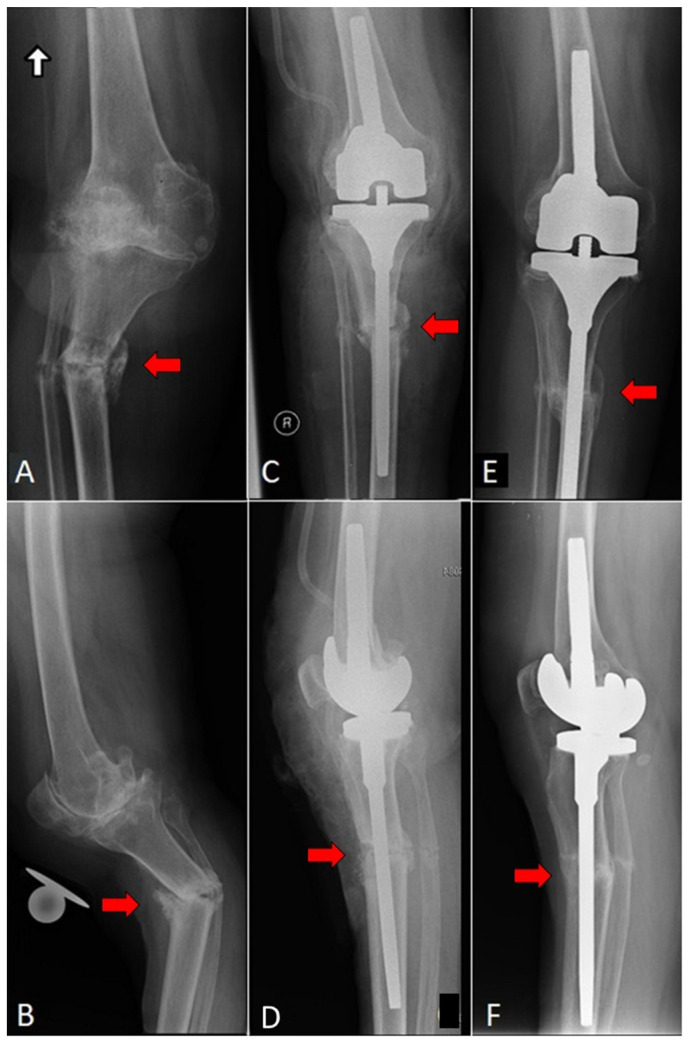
(A) AP and (B) lateral radiograph of the patient with 1/3 proximal tibial and fibular shaft fractures (pseudarthrosis) and coexisting severe knee osteoarthritis. Early postoperative radiographs (C) AP and (D) lateral (Scorpio TS, Stryker, Mahwah, NJ, USA). CCK knee implant with long tibial stem was used. (E) AP and (F) lateral radiographs of the knee performed.
